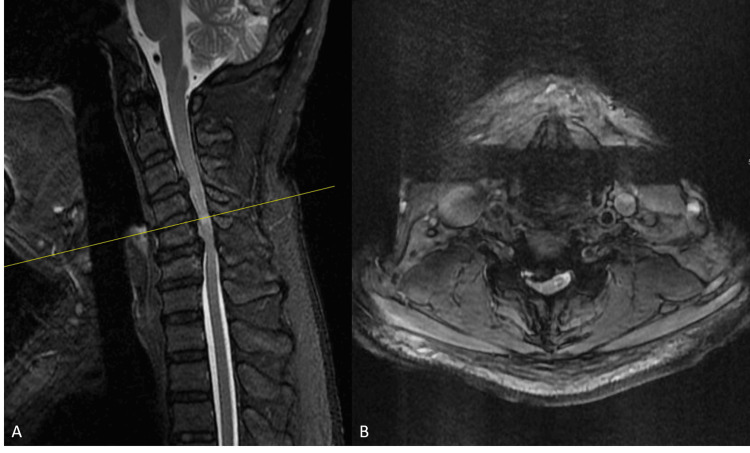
Preoperative sagittal (A) and axial (B) MRI showing multilevel cervical cord compression, C4-C5 myelomalacia, and mild kyphosis
